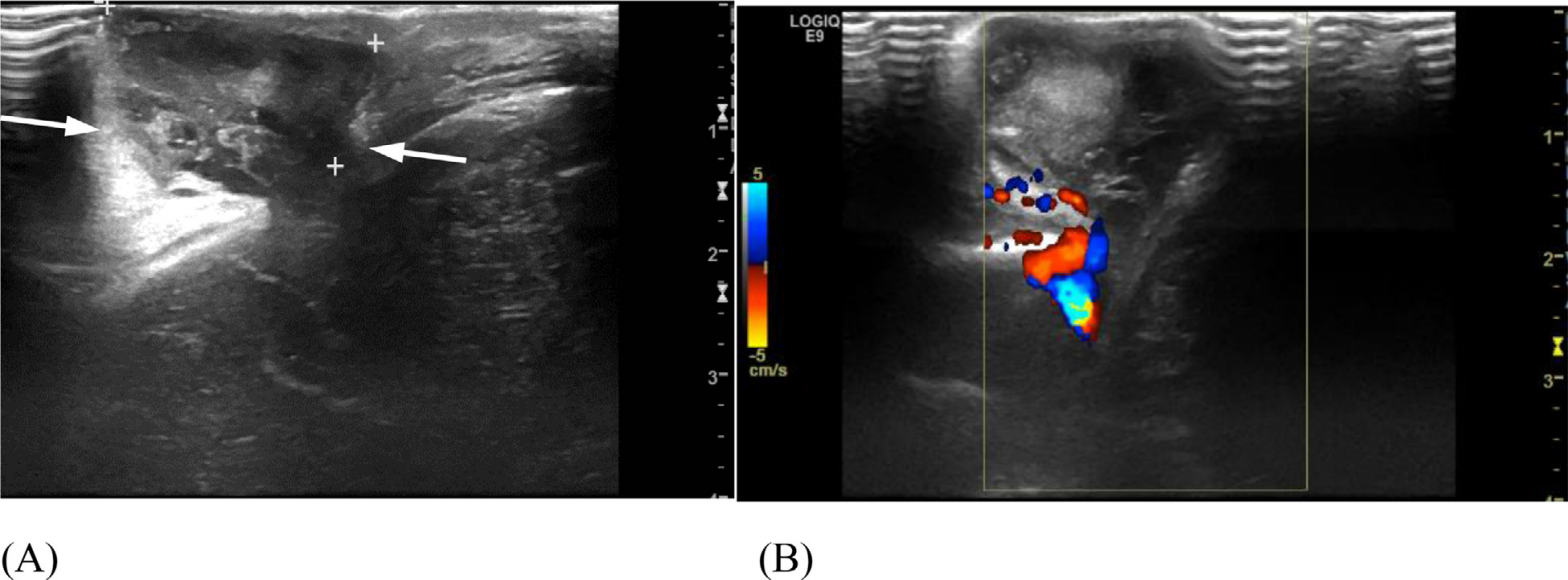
(Case 2) Head ultrasound without (A) and with (B) color Doppler demonstrates a skull defect and central echogenic debris herniating outside the calvarium. Color Doppler flow shows a falcine sinus extending into the extracalvarial mass, consistent with an atretic posterior cephalocele.
